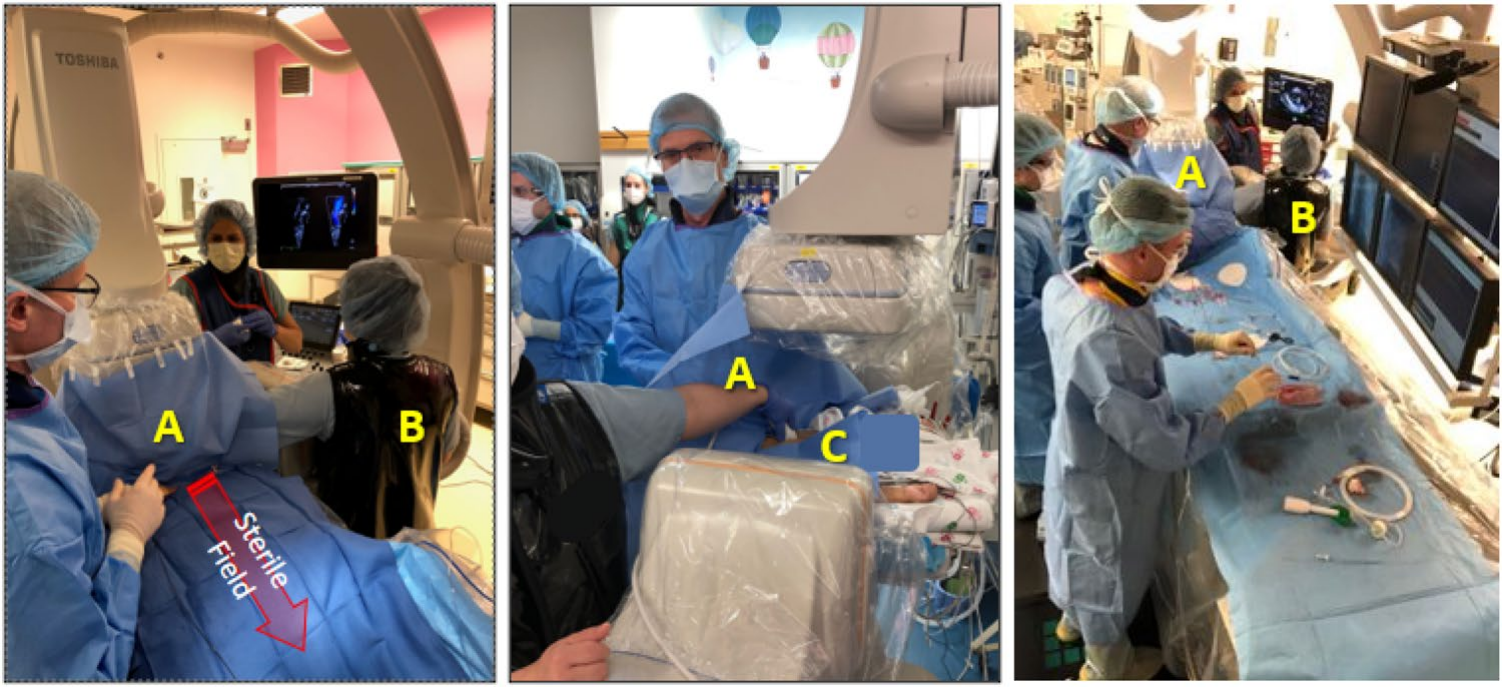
(A) Drape extended from patient abdomen to AP camera, dividing sterile and non-sterile fields. (B) Sonographer in position to allow comfortable access to patient while maintaining sterile field. (C) Drapes applied to allow subxiphoid, apical, and parasternal views
